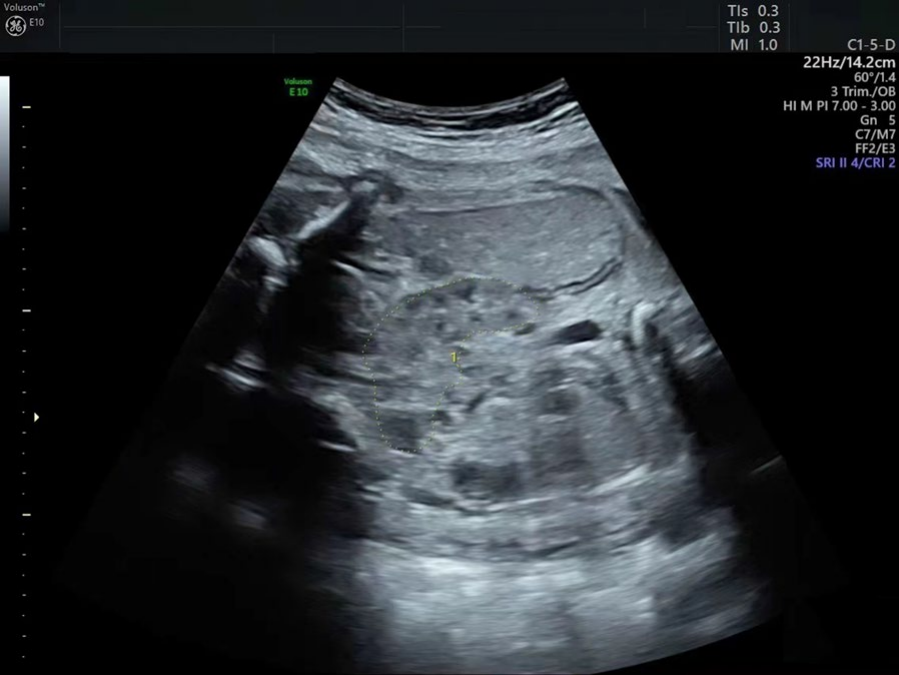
Ultrasound image of the horseshoe kidney
